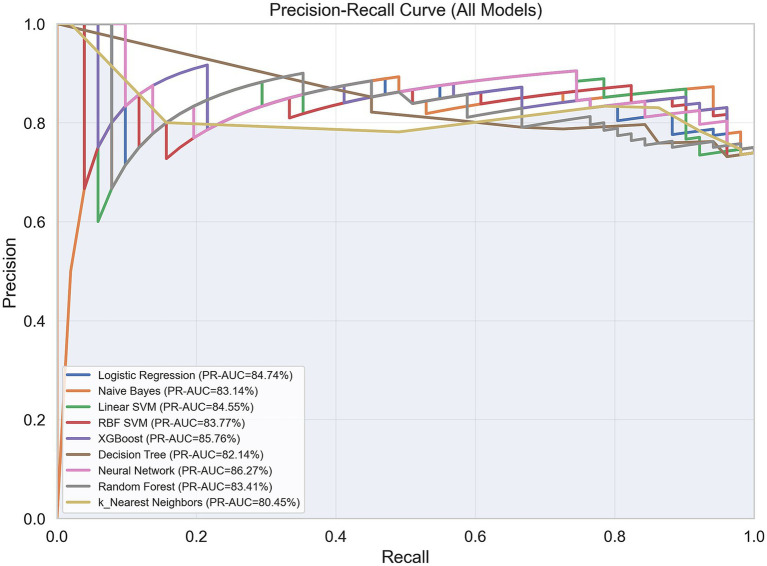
Precision–recall (PR) curves of the machine learning models for predicting PET/CT usefulness in patients with FUO/IUO.
